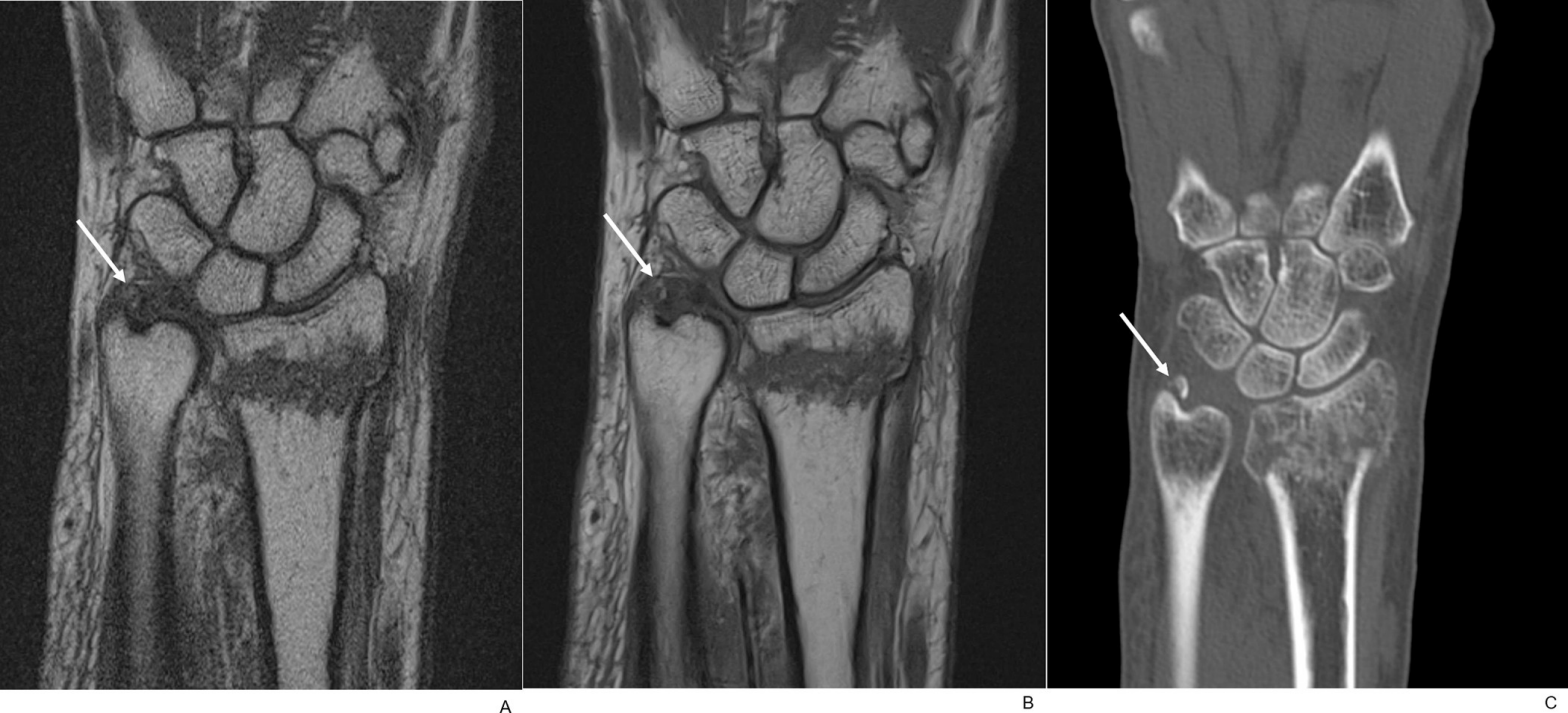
Associated ulnar styloid process fracture. (A) Coronal T1-weighted TSE_S_ image of a 60-year-old female with a distal radius fracture demonstrated suspected fragmentation of the ulnar styloid process with indistinct cortices. (B) However, a small bony fragment with distinct cortices and internal fatty marrow was well delineated on the corresponding TSE_DL_ image. (C) Coronal CT image clearly showed ulnar styloid process fracture with bone fragments.
